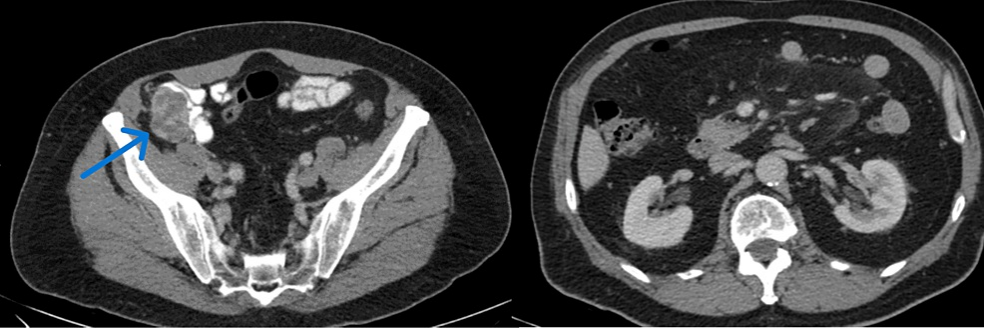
Left: Computed tomography imaging of the mass (axial plane). Right: Computed tomography imaging of the kidneys, without evidence of mass (axial plane). The arrow shows the enhanced mass located in the right iliac fossa, with a diameter of 4.3 cm.
